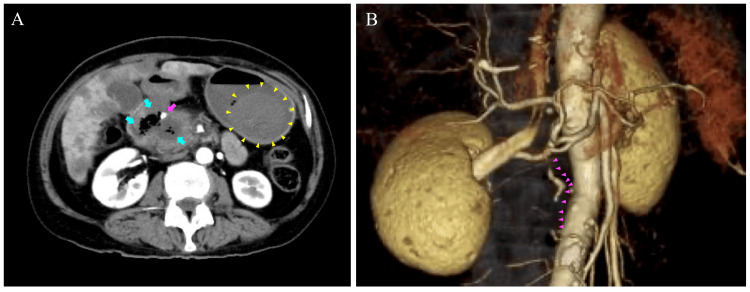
Contrast-enhanced CT and three-dimensional CT angiography immediately after hematemesis The slightly high-density area when compared to the other areas in the gastrointestinal tract on contrast-enhanced computed tomography (CT), immediately after hematemesis, is considered as gastrointestinal hemorrhage or blood clots. The parenchymal border between the duodenum and pancreatic head is indistinct, and intraluminal air is observed within this region (cyan arrows). These findings are suggestive of either duodenal or pancreatic head wall disruption, with possible exposure of the tumor surface into the duodenal lumen. Contrast-enhanced blood vessels can be identified in proximity to the tumor (magenta arrow). No obvious contrast leakage can be observed in the gastrointestinal tract or other areas (A). Three-dimensional CT angiography reveals signs of vasospasm in the pancreatoduodenal arcade, including irregular vessel calibers and filling defects, consistent with post-hemorrhagic vasoconstriction (magenta arrowheads) (B).
